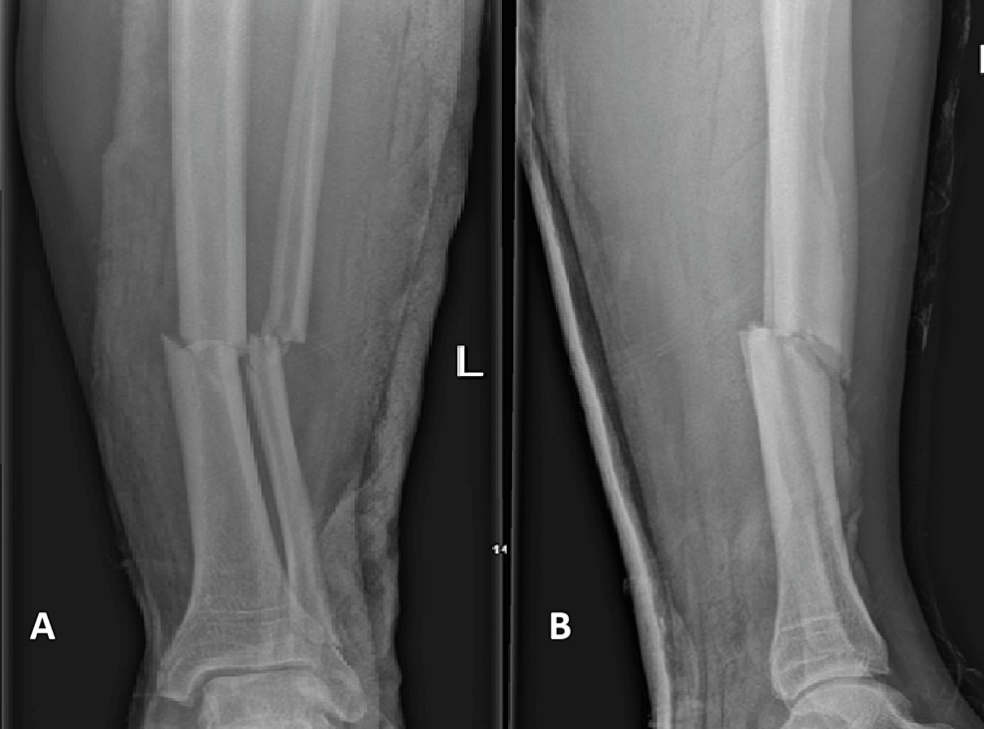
Anteroposterior (A) and lateral (B) radiographs of one of the cases in this study with a tibia/fibula fracture on presentation
